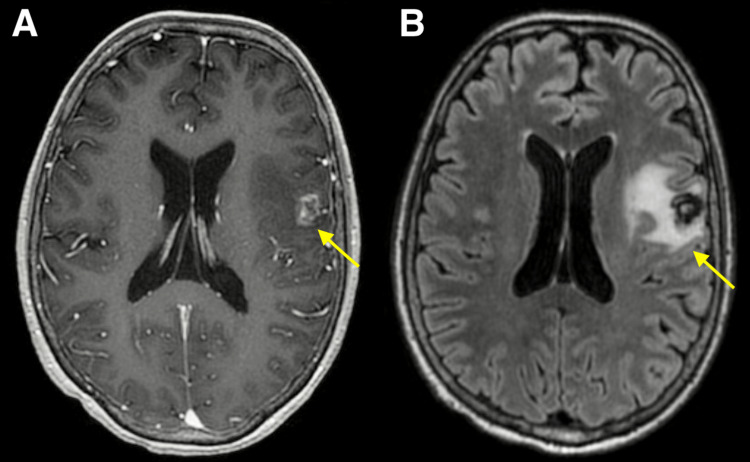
MRI 30 months after Gamma Knife stereotactic radiosurgery. Axial contrast-enhanced T1-weighted (A) and T2-weighted FLAIR brain MRI (B) obtained 30 months after Gamma Knife stereotactic radiosurgery demonstrating a 1.2 cm enhancing lesion in the left frontal lobe at the site of previously treated metastasis with adjacent vasogenic edema. FLAIR: Fluid-attenuated inversion recovery
